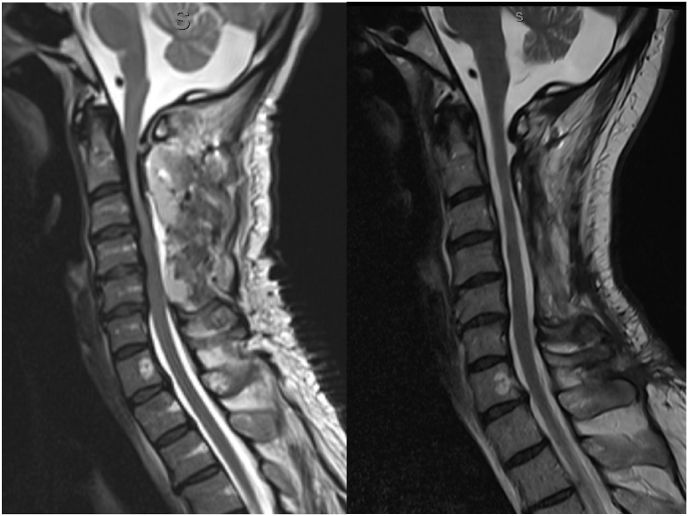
MR-imaging (T2-weighted) on third postoperative day on the left and twelve months after surgery. A regression of the T2-hypointense thickening of the dura, especially at the level C2, is noted after treatment with methylprednisolone and rituximab.
